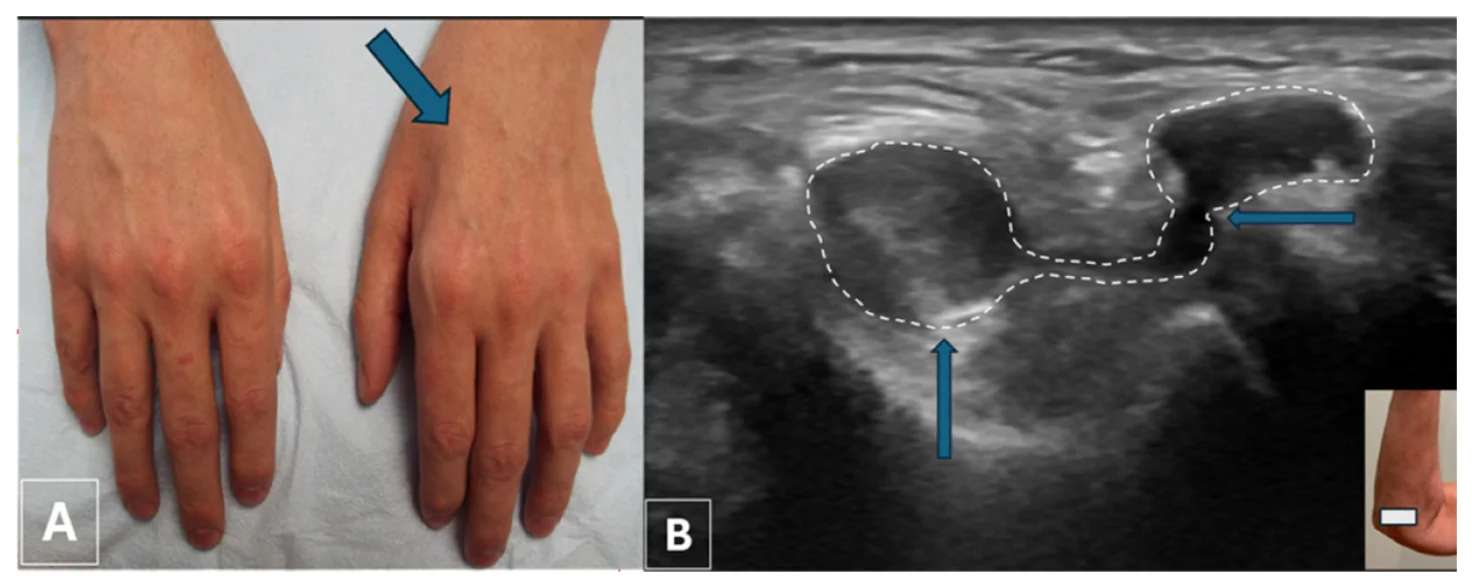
Case #3: (A) Arrow points to atrophy of the FDI muscle of the left hand. (B) Ultrasound short-axis view at the elbow showing a markedly enlarged ulnar nerve (vertical arrow) from an intraneural cyst, communicating (outlined by a dotted line) with a nearby extraneural cyst (horizontal arrow).
